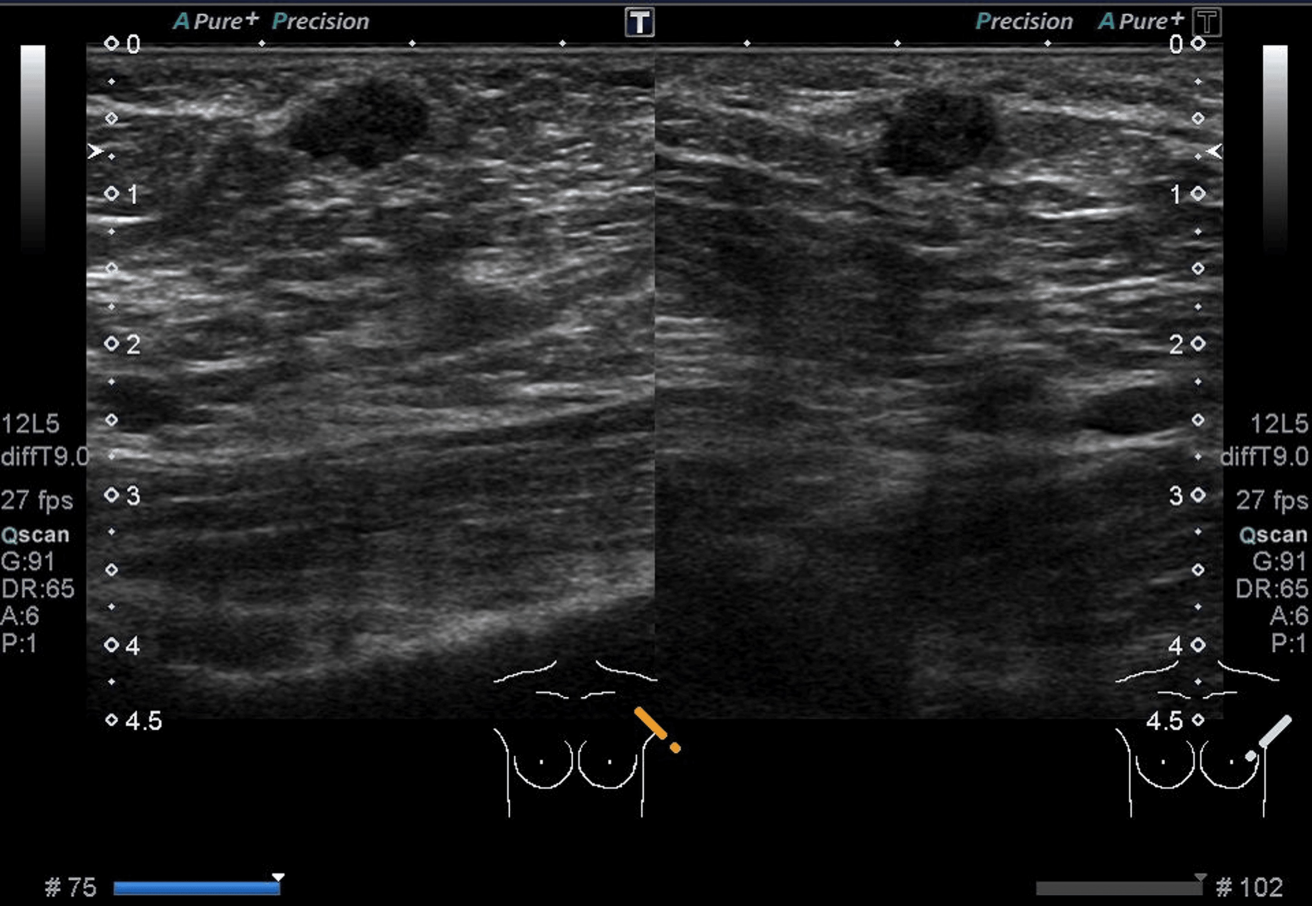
Sonography showing an oval, homogenous, relatively well-defined, hypoechoic mass in the left axilla, measuring 0.9 cm.
